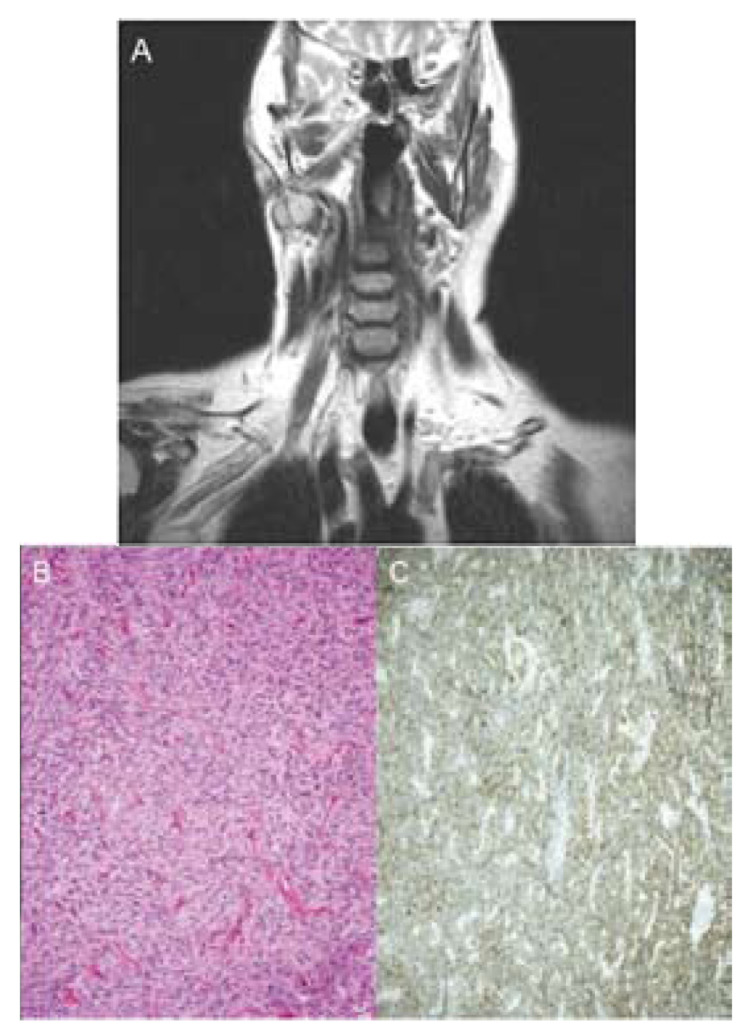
A. Contrast-enhanced T2-weighted magnetic resonance image. Note a well-defined tumor in the right parotid gland. It shows high signal intensity with homogeneous enhancement; B. Tumor cells arranged in a storiform pattern. C. They show reactivity with bcl-2.
